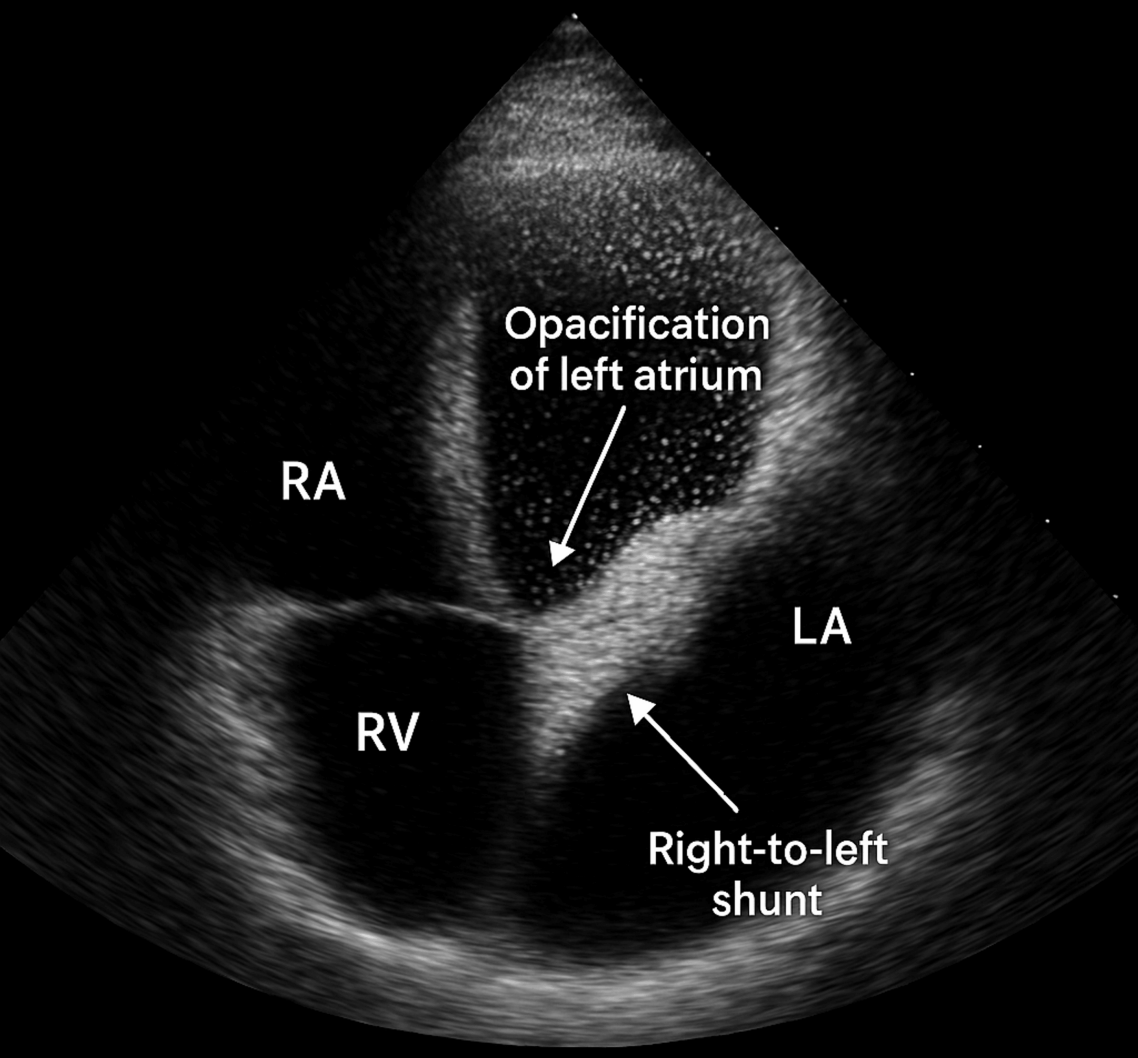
Transthoracic echocardiography showing opacification of LA and right to left shunt RA: right atrium, RV: right ventricle, LA: left atrium
